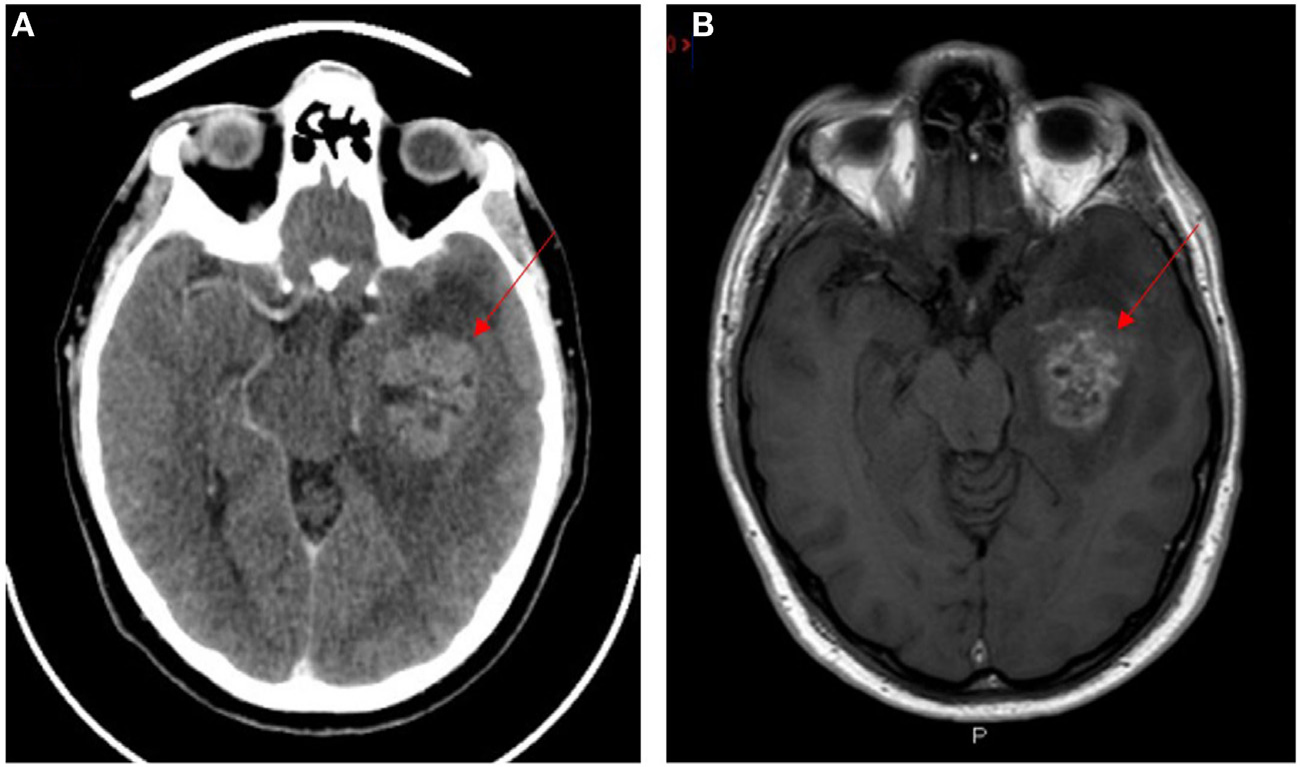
CT (A) and T1 weighted MRI (B) scans with contrast of a patient with glioblastoma multiforme. Lesion indicated by red arrows.
